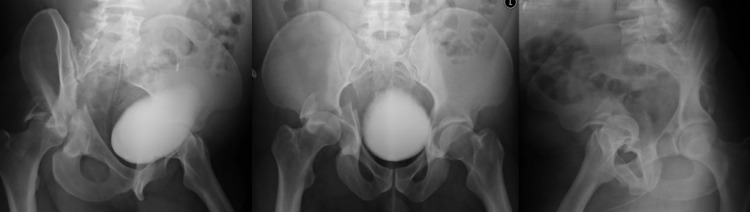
Obturator oblique, anteroposterior, and iliac oblique radiographs of an acetabular fracture
